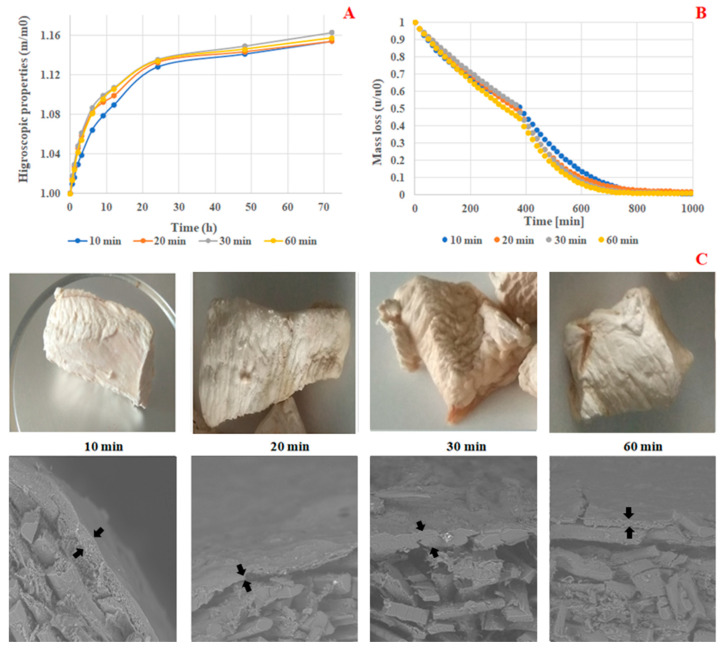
Hygroscopicity kinetics (A), relative humidity (B), and macro/microstructure, ×500 (C), of coated freeze-dried chicken meat treated with ultrasound.
